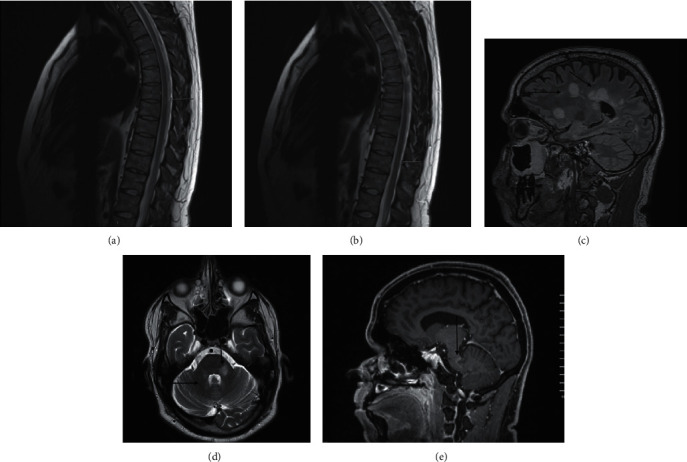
((a), (b)) There are at least 2 definite high signal intensity plaques on the sagittal T2W1 seen at D6-7, D9-10 levels (arrow). ((c), (d)) Numerous bilateral variable-sized, different-shaped plaques of high signal intensity on T2W1/FLAIR seen mainly in the deep white matter at periventricular location and centrum semiovale, perpendicular callososeptal lesions, cerebellar peduncles, and cerebellar white matter. (e) Incomplete ring enhancement lesions seen in the left deep periventricular frontal lobe and left cerebellar peduncle.
